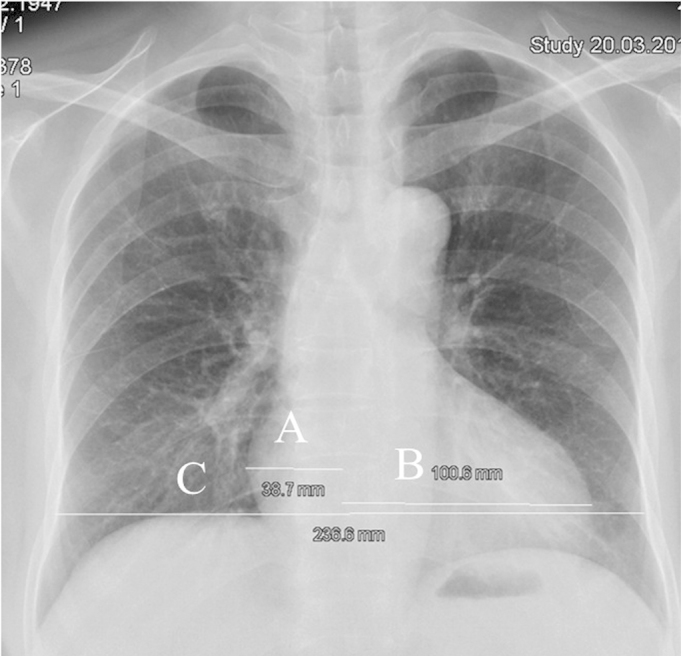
Method for determining CTR from chest radiography. The midline was defined as a vertical line drawn through the spinous processes. (A) The maximum distance from the midline to the right cardiac border was added to (B) the maximum distance from the midline to the left cardiac border. Transverse diameter of the cardiac silhouette = A+B. (C) The distance between the internal margins of the ribs at the level of the right hemidiaphragm. CTR = (A+B)/C. CTR, cardiothoracic ratio.
